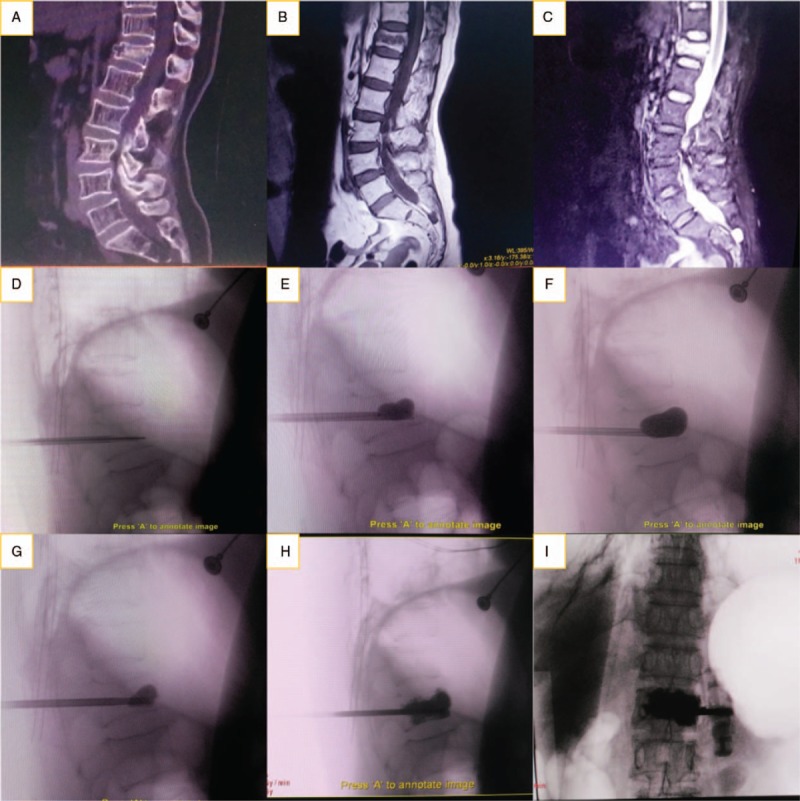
(A–C) MRI images showed a patient diagnosed with a T12 vertebral compression fracture. PKP was performed with directional balloon dilation technology. Preoperative CT, T1, and T2 fat suppression sequences of preoperative MRI showing that T12 was fresh OVCF and the fracture area was in the middle. (D–F) Images showing the needle located in the lower part of the vertebra, near the lower endplate, working channel adjusted to move the notch facing upward. In the process of balloon dilation, it expanded in an upward direction. (G–I) Images showing the cement first diffusing evenly upward and the fracture and surrounding area fully filled finally. CT = computed tomography, MRI = magnetic resonance imaging, OVCF = osteoporotic vertebral compression fracture, PKP = percutaneous kyphoplasty.
